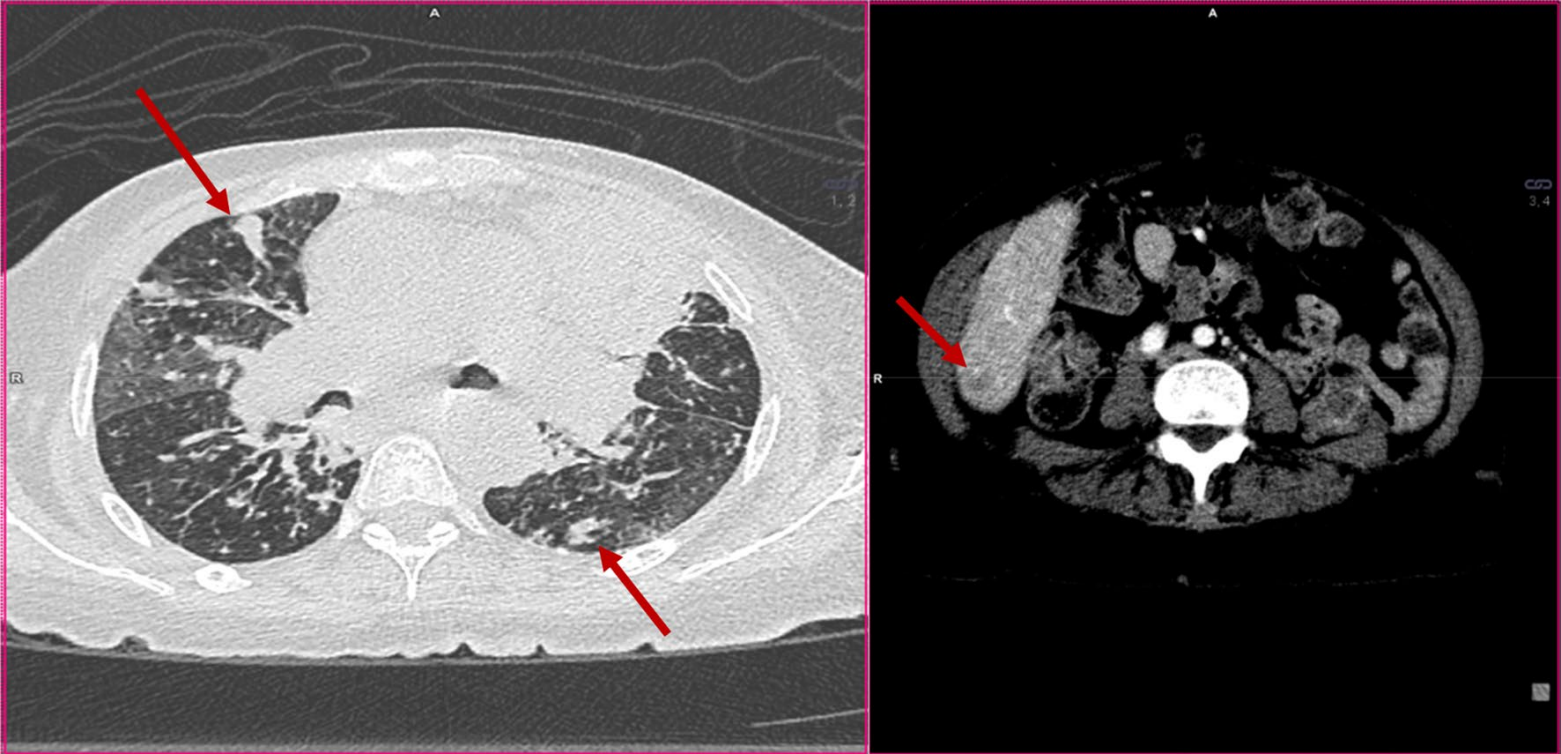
CT chest confirmed progression of the malignant disease, with innumerable bilateral nodular lesions, and new hypodense lesion in segment 6 of the liver.
